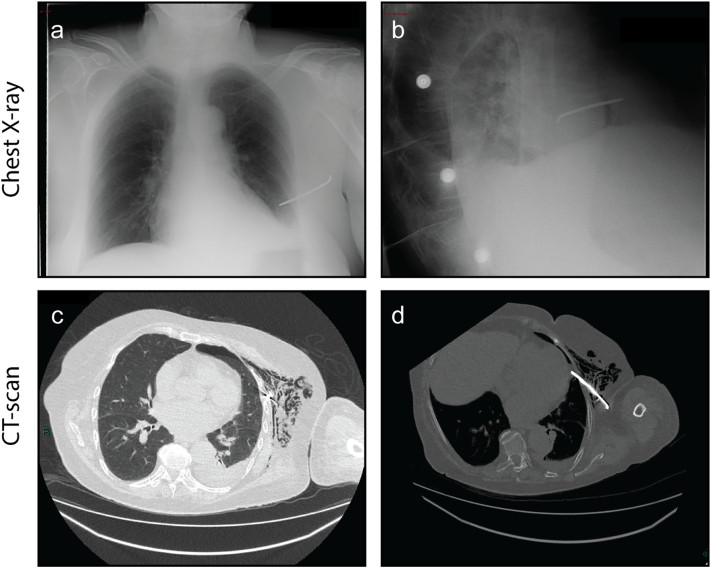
A–B: AP and lateral X-thorax. C: CT-thorax in pulmonary setting, ventrally a mild pneumothorax with subcutaneous emphysema. D: CT-thorax in bone setting, the K-wire located intrathoracically.
